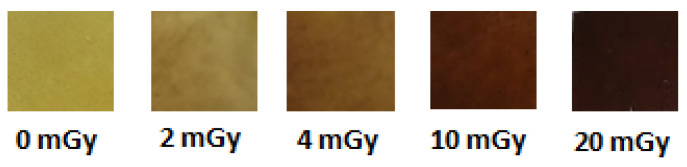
Direct perceived color change of PVA/Ag^+^/NBT films irradiated with different X-ray doses.
